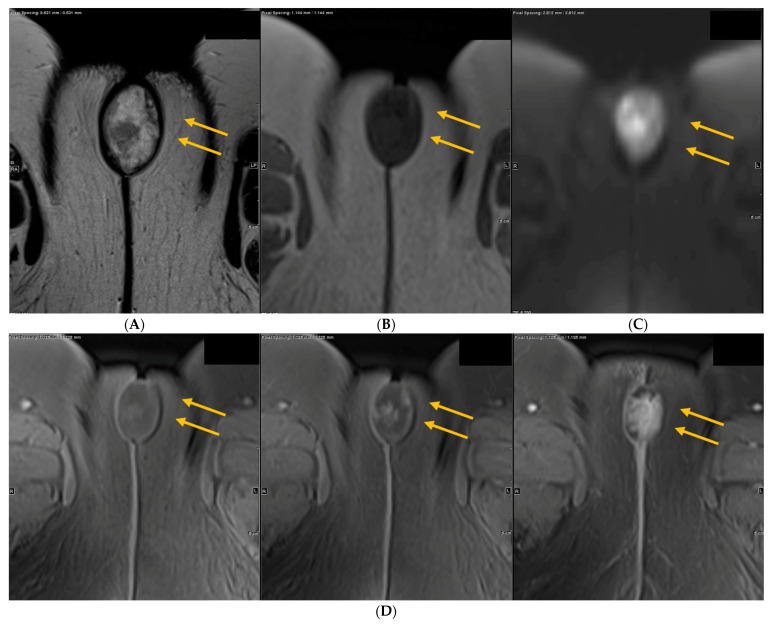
Imaging findings from MRI of pelvis. (A) Axial T2 image of the pelvis shows a heterogenous, well circumscribed T2 hyperintense clitoral mass with T2 hypointense margin with internal low signal (yellow arrows). (B) On axial T1, the lesion is hypointense, typical of most malignancies (yellow arrows). (C) On axial diffusion weighted imaging (DWI) with B = 800, the clitoral mass is bright (restricting) suggesting high cellularity (yellow arrows). (D) On axial dynamic T1 post contrast, the clitoral mass progressively enhances.
